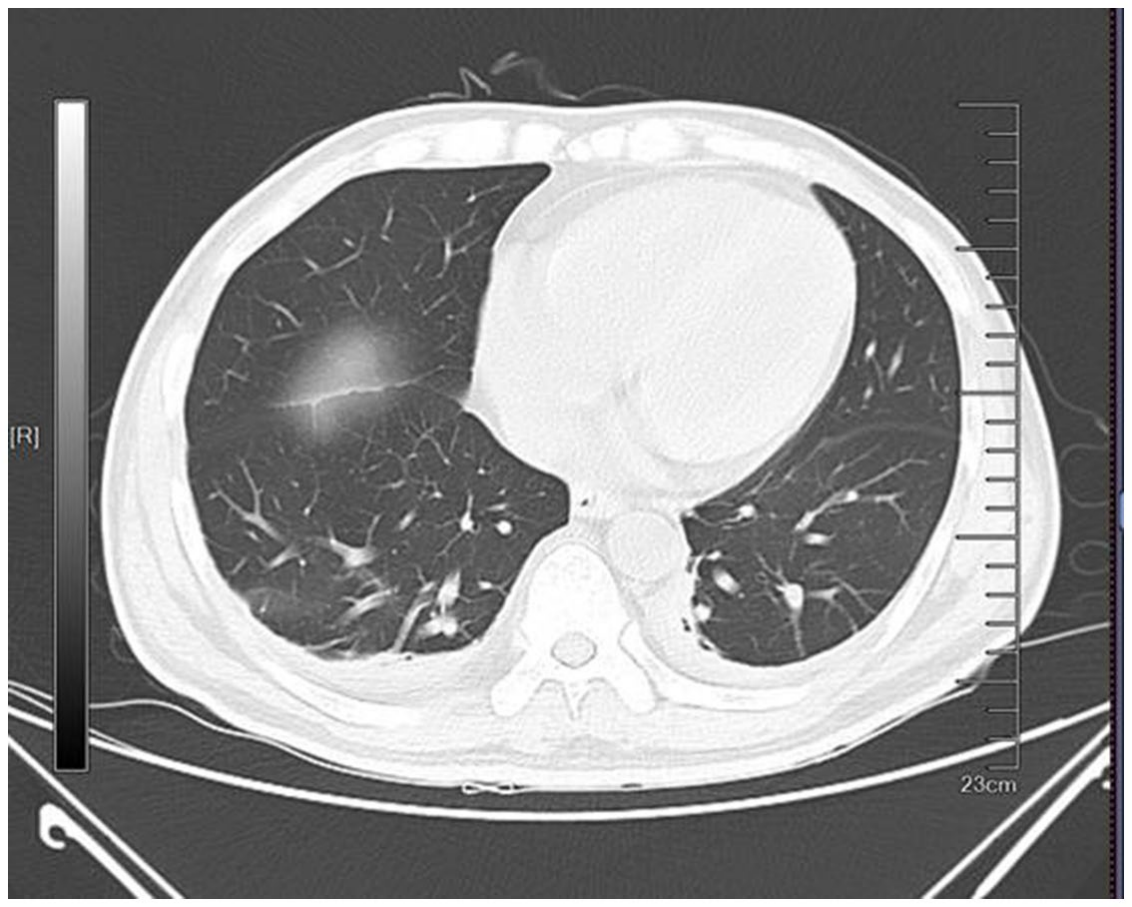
CT image of the patient’s chest. The CT showed bilateral pulmonary exudative changes and bilateral pleural effusion.
